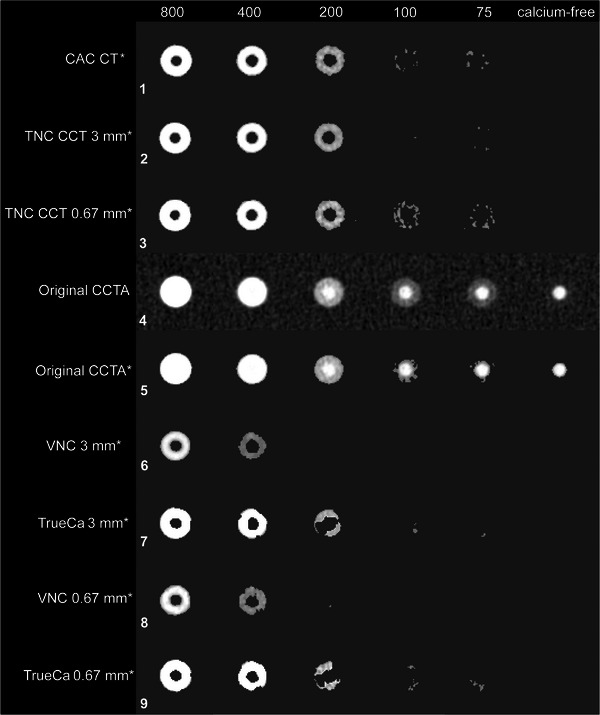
Example images comparing coronary artery calcium (CAC) scoring CT, true non contrast coronary CT (TNC CCT), coronary CT angiography (CCTA) and two virtual non‐contrast (VNC) methods. The top three rows show in vitro CAC CT and TNC CCT (3 mm and 0.67 mm) of calcifications with varying coronary artery calcium (CAC) densities in mg/cc. The fourth to ninth row show images from contrast‐enhanced CCTA. In the sixth and eighth row, the CT values of calcifications were suppressed by the calcium non‐specific VNC method, resulting in undetected calcifications with medium and low density (200, 100, and 75 mg CaHA/cc). In contrast, the seventh and ninth row applies the calcium‐preserving TrueCa approach, which preserves CT values of CAC, improving detection of calcifications of all densities. An asterisk (*) is used to indicate that calcium thresholding is applied at 130 Hounsfield units (HU) (WL, 70 HU; WW, 600 HU).
